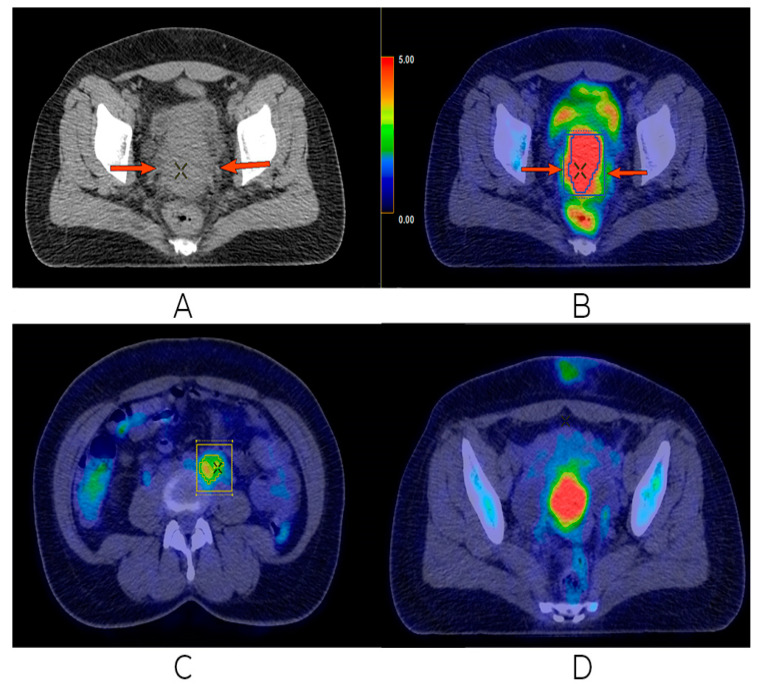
Second FDG PET/CT. Discrete progression in the uterine cervical tumor (A,B), as well as progression in the left aortic retroperitoneal adenopathy (C), but with remission of the left external iliac nodules (D).
